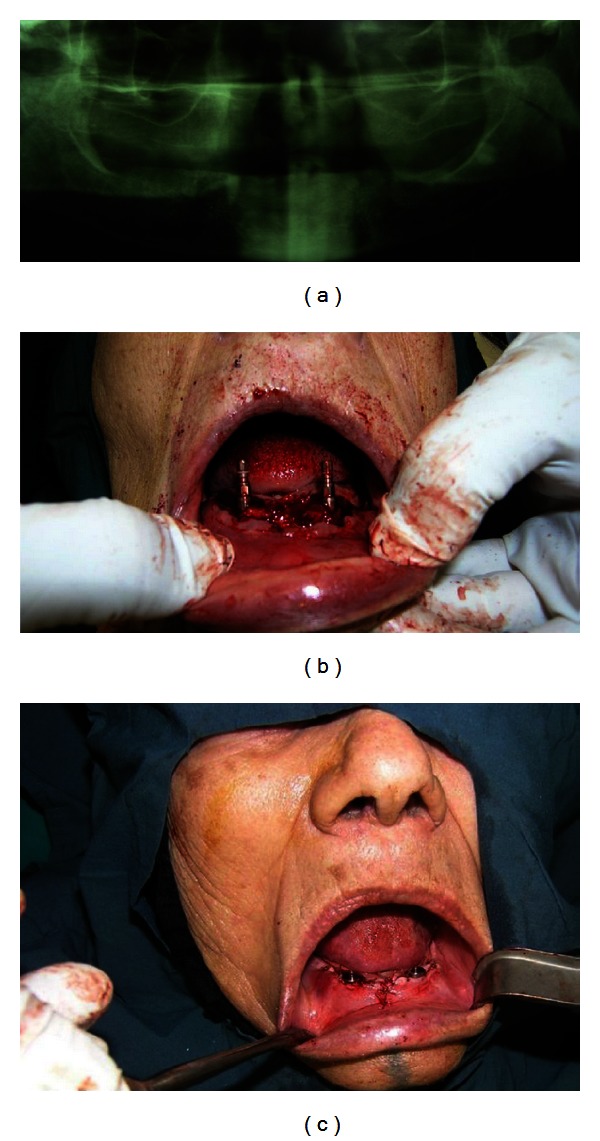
(a) Patient number two, panoramic X-ray before surgery. (b) Patient number two, surgery day photo. (c) Patient number two, after surgery photo.
